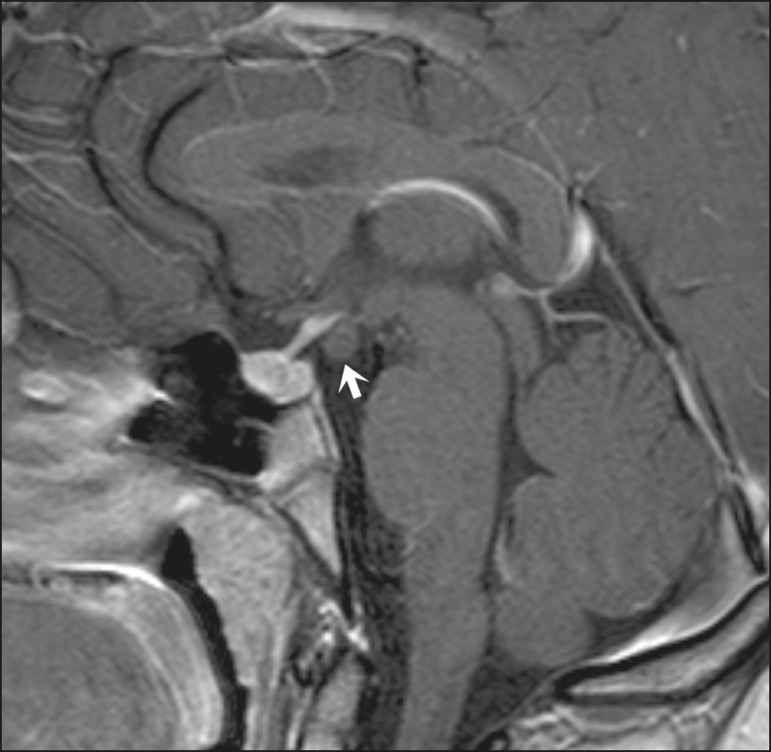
Contrast-enhanced sagittal T1-weighted MRI sequence showing a
